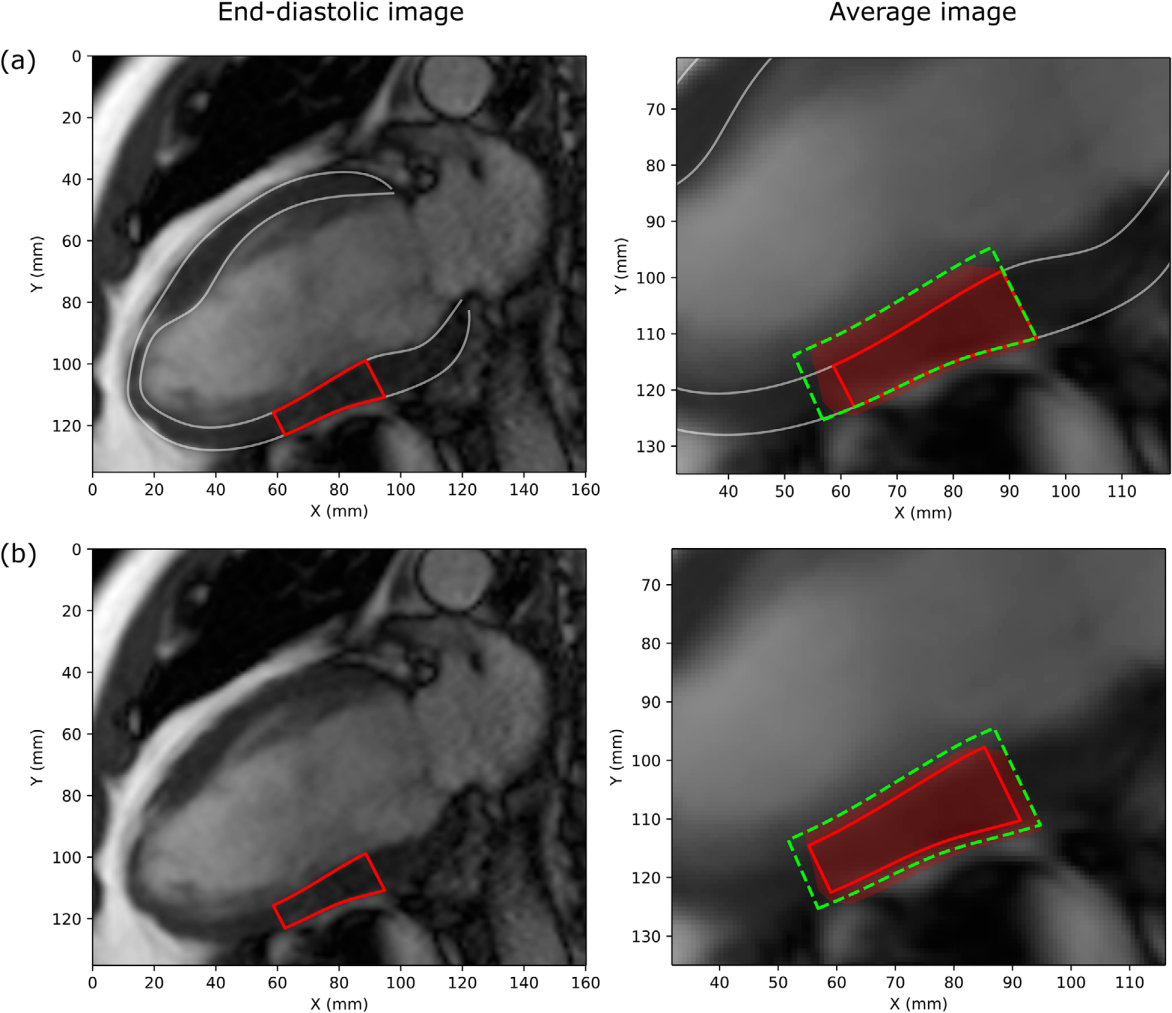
The applied ITV margin will depend on the imaging method. In figure (a), the LV and target are defined on the end‐diastolic image and the proposed ITV is shown for the case where the entire LV is matched on the average image (e.g., CBCT). Figure (b) shows an example where the target itself is used for matching and the ITV is composed of halved epicardial and endocardial radial/longitudinal displacements in all directions. The average image in this figure was generated by averaging all CMR phase images to approximate a CBCT (and the associated image blur) typically used during treatment. CBCT, cone beam computed tomography; CMR, cardiovascular magnetic resonance; dashed green outline, proposed ITV based on mean HFrEF displacement data; ITV, internal target volume; LV, left ventricle; red outline, target; red shaded area, actual area covered by target over cardiac cycle.
